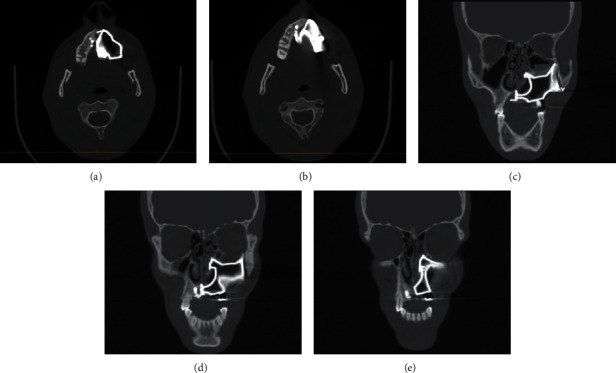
Computed tomography after 3 years of follow-up: (a, b) axial cuts and (c–e) coronal cuts.
